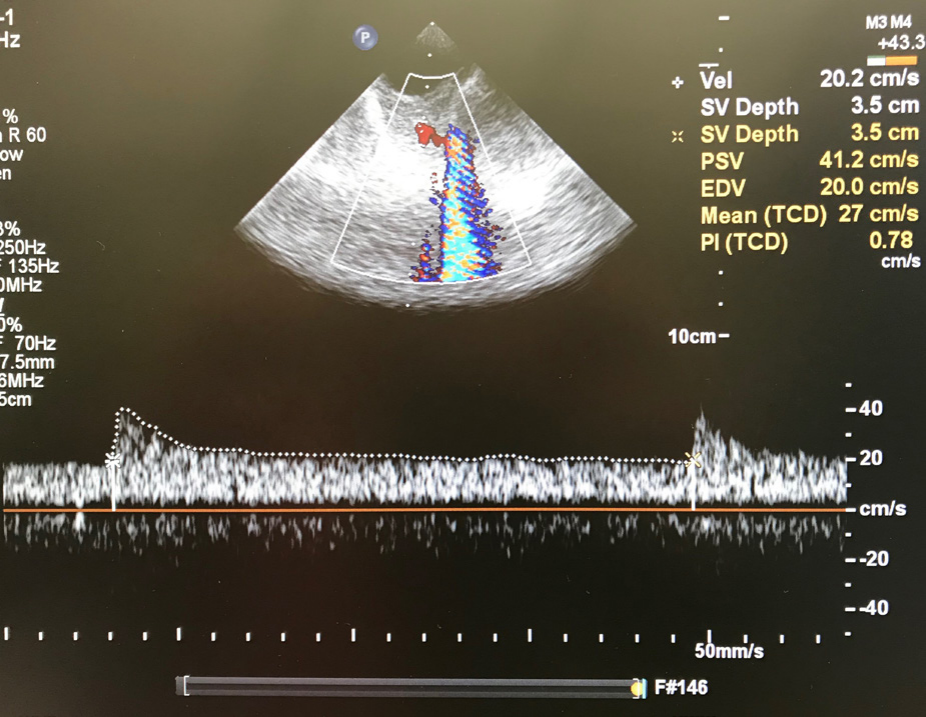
Colour Doppler imaging of the ophthalmic artery, 15 minutes after anaesthesia induction. Ophthalmic artery (OA) mean velocity: 27 cm sec^−1^.
